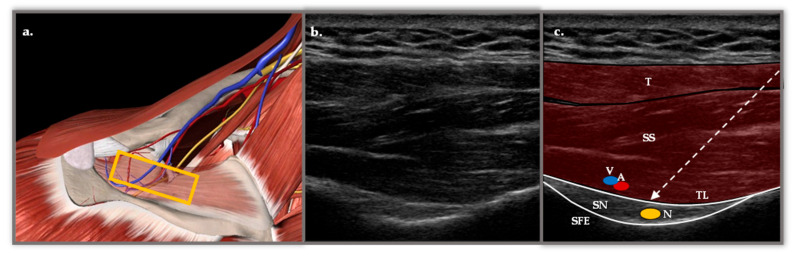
(a) Anatomical representation in Essential Anatomy 5 of the approach to the SSBN in the supraspinatus fossa. The muscular plane of the trapezius (T) has been removed and the supraspinatus (SS) has been faded, revealing the depth of the suprascapular nerve (N), vein (V) and artery (A) in the scapular notch (SN). The yellow rectangle represents the longitudinal position of the probe. (b) Longitudinal ultrasound section with a 6 and 15 MHz flat probe in supraspinatus fossa. (c) Representation of the most important structures with two muscle layers corresponding to the T and the SS. Below, two hyperechoic structures appear, the transverse ligament (TL) and the SE, which form the roof and floor of the fossa through which the N passes. The TL separates the V and A structures from the N. The dashed arrow represents the course of the needle with a posterior approach from medial to lateral.
